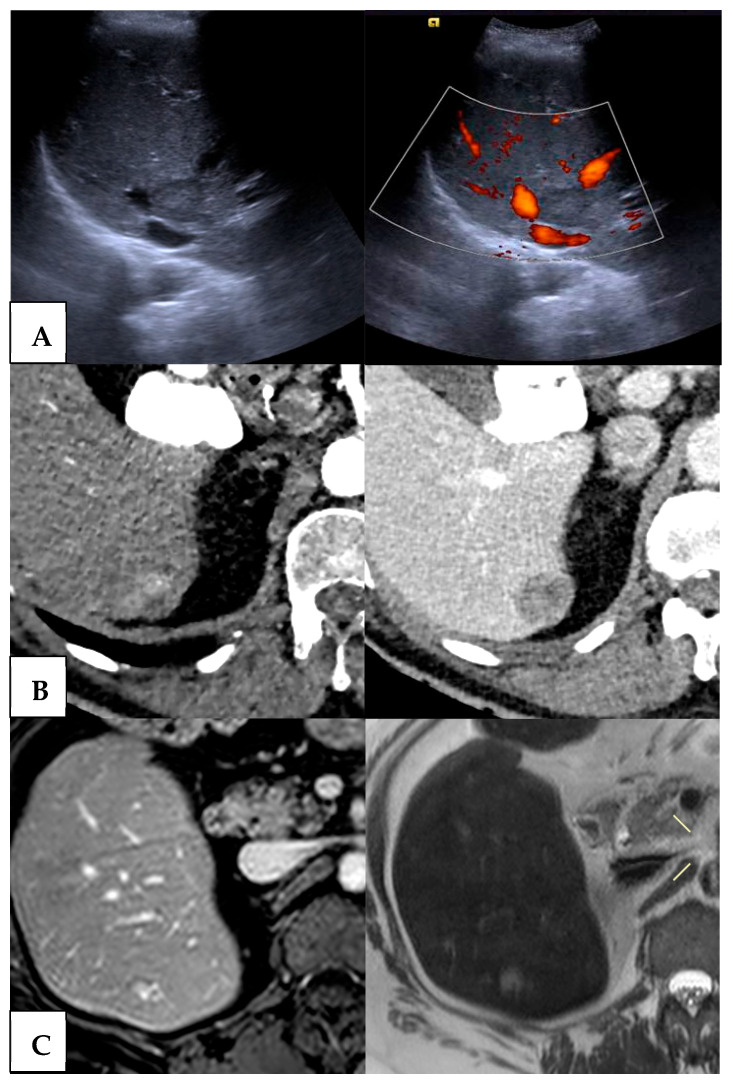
Representative images of liver lesions initially labeled as atypical hemangiomas in radiology reports, later diagnosed as malignant. (A) Ultrasound images, including color Doppler, of metastasis of gastrointestinal origin. (B) CT scan showing arterial and portal phase images of a neuroendocrine tumor (NET). (C) MRI scan showing T1 portal phase and T2-weighted images of liver metastases from breast cancer.
